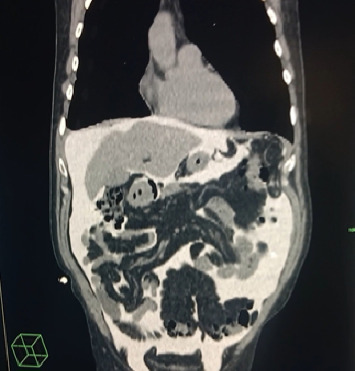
Peritoneal CT scan after restoration of the peritoneal method.
